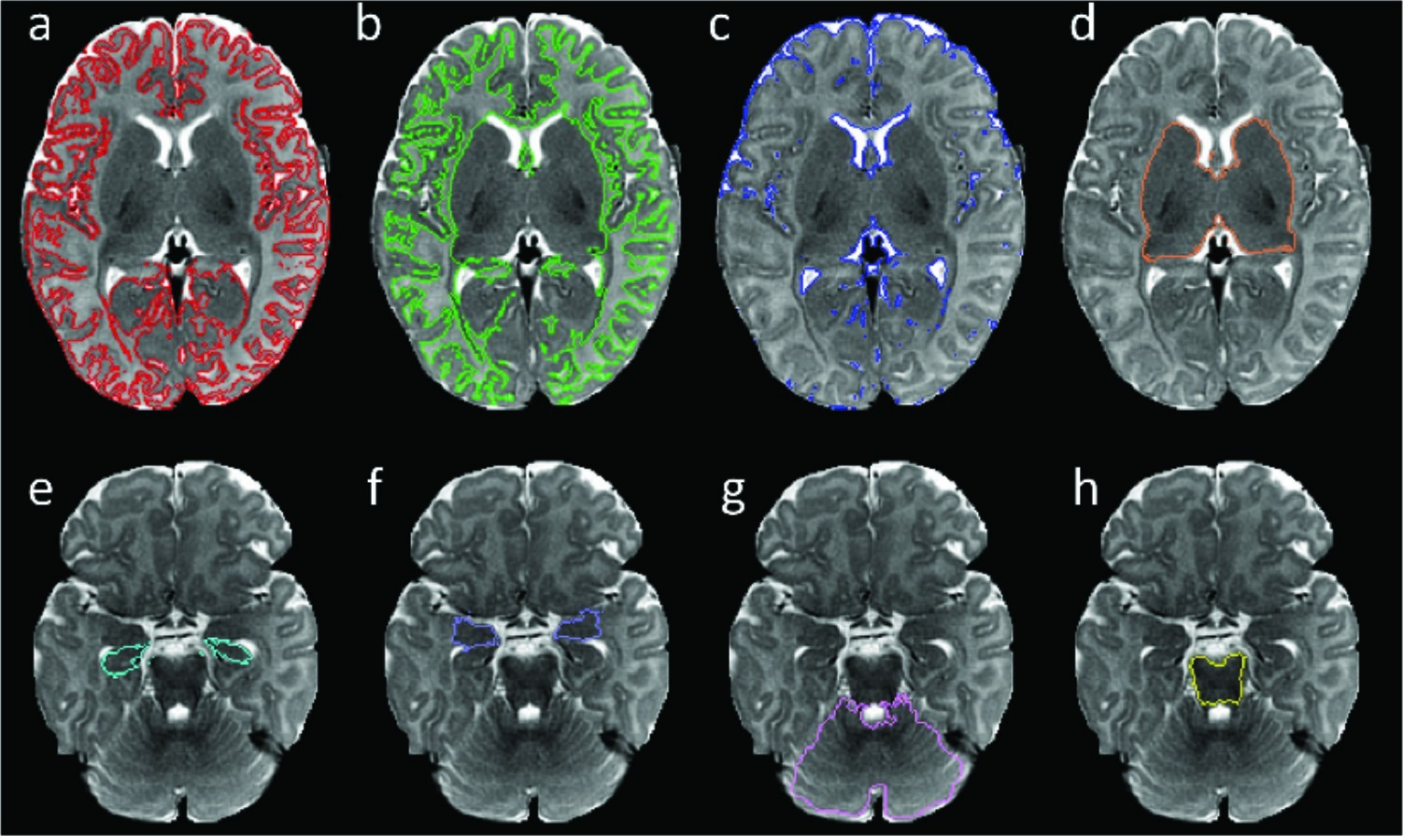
Representative axial slices of a T2-weighted MR image obtained from a ZIKV-exposed subject. The colored contours highlight the 8 ROIs segmented by MANTiS as: (a) cortical grey matter in red, (b) white matter in green, (c) cerebrospinal fluid in dark blue, (d) nuclear deep grey matter in orange, (e) hippocampus in light blue, (f) amygdala in purple, (g) cerebellum in pink, and (h) brainstem in yellow.
